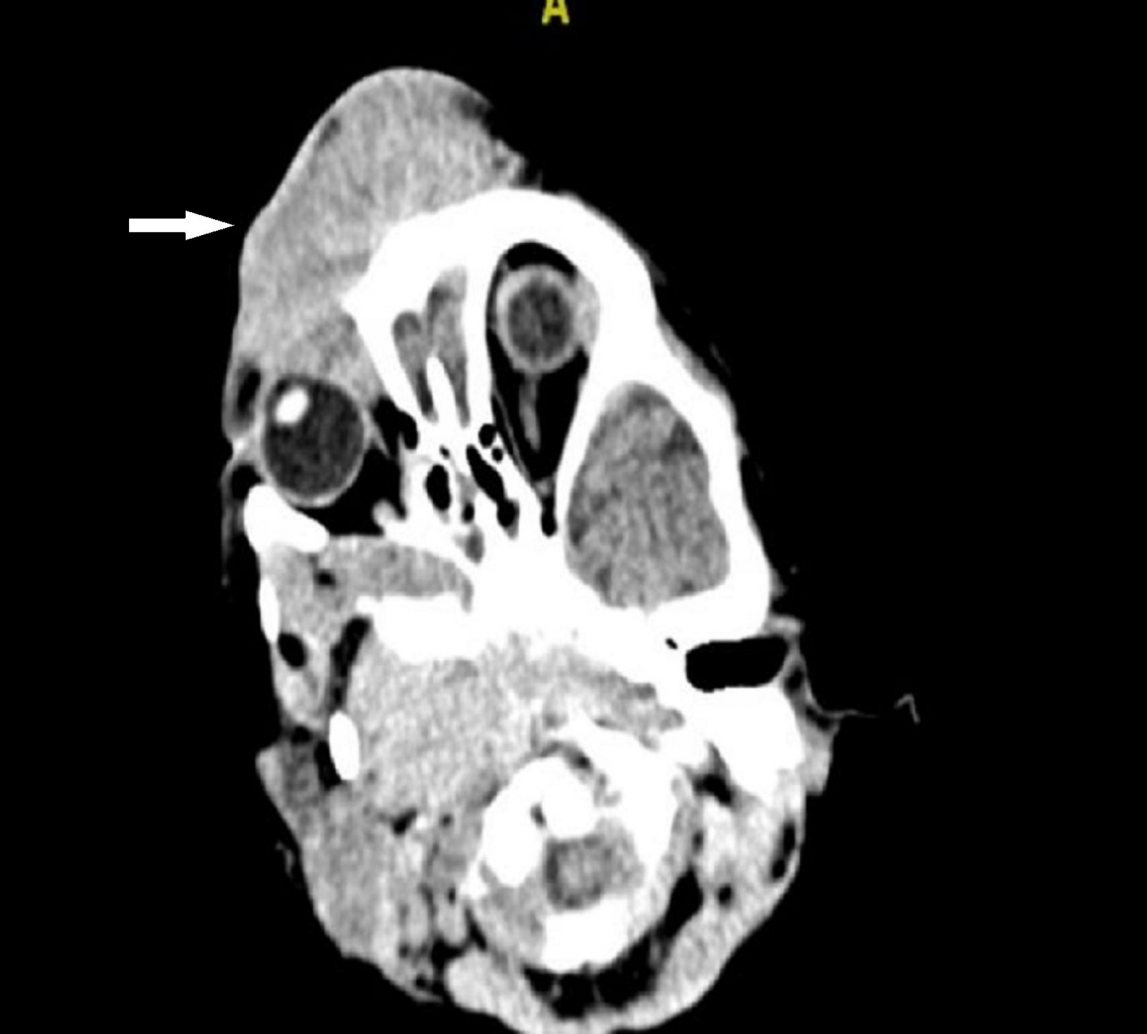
Computed tomography scan of the brain showing multiple well-defined enhanced lesions at the frontal scalp with the largest lesion at the anterior glabella. The lesion extended into the bilateral orbital cavities and caused lateral displacement of the bilateral orbits. The bilateral globes were normal.
